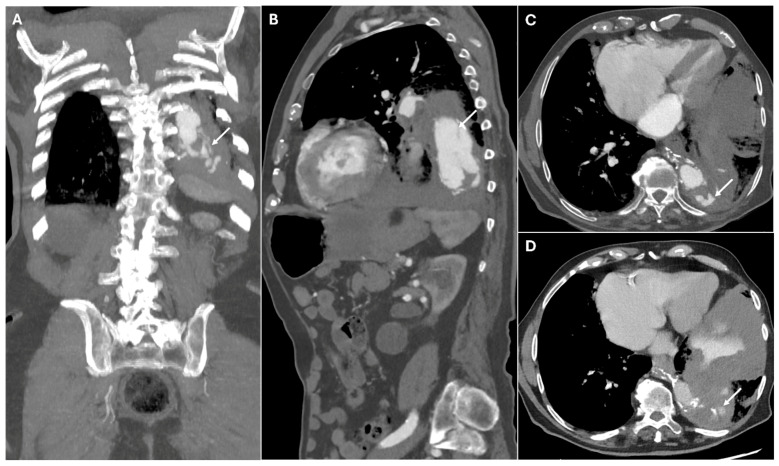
CT images of graft dehiscence. Panels (A,B) show axial and sagittal CTA images demonstrating dehiscence of the distal anastomosis of the thoracic aortic graft (white arrows). The disrupted graft connection results in direct communication between the vascular lumen and the surrounding mediastinal structures, revealing contrast extravasation into the left lung parenchyma (Panels (C,D)—white arrows).
